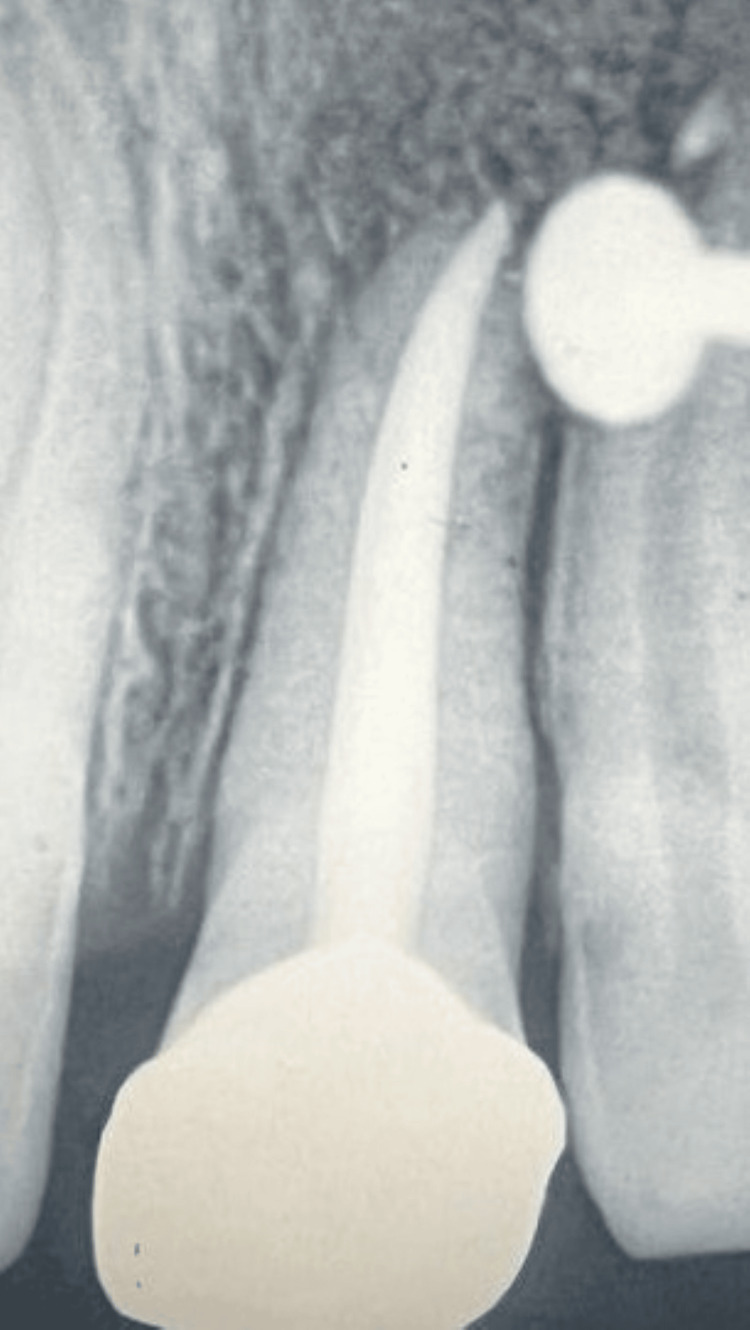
Radiograph taken during the follow-up session at 20 weeks; a nosepin visible as an artifact.
